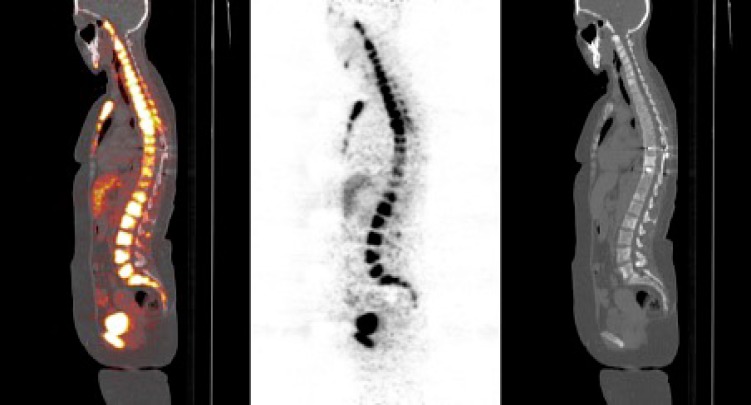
Galium-68 DOTATATE positron emission tomography/computed tomography images of a 47-year-old female patient with primary pancreatic grade 2 neuroendocrine tumor showing widespread bone metastases
